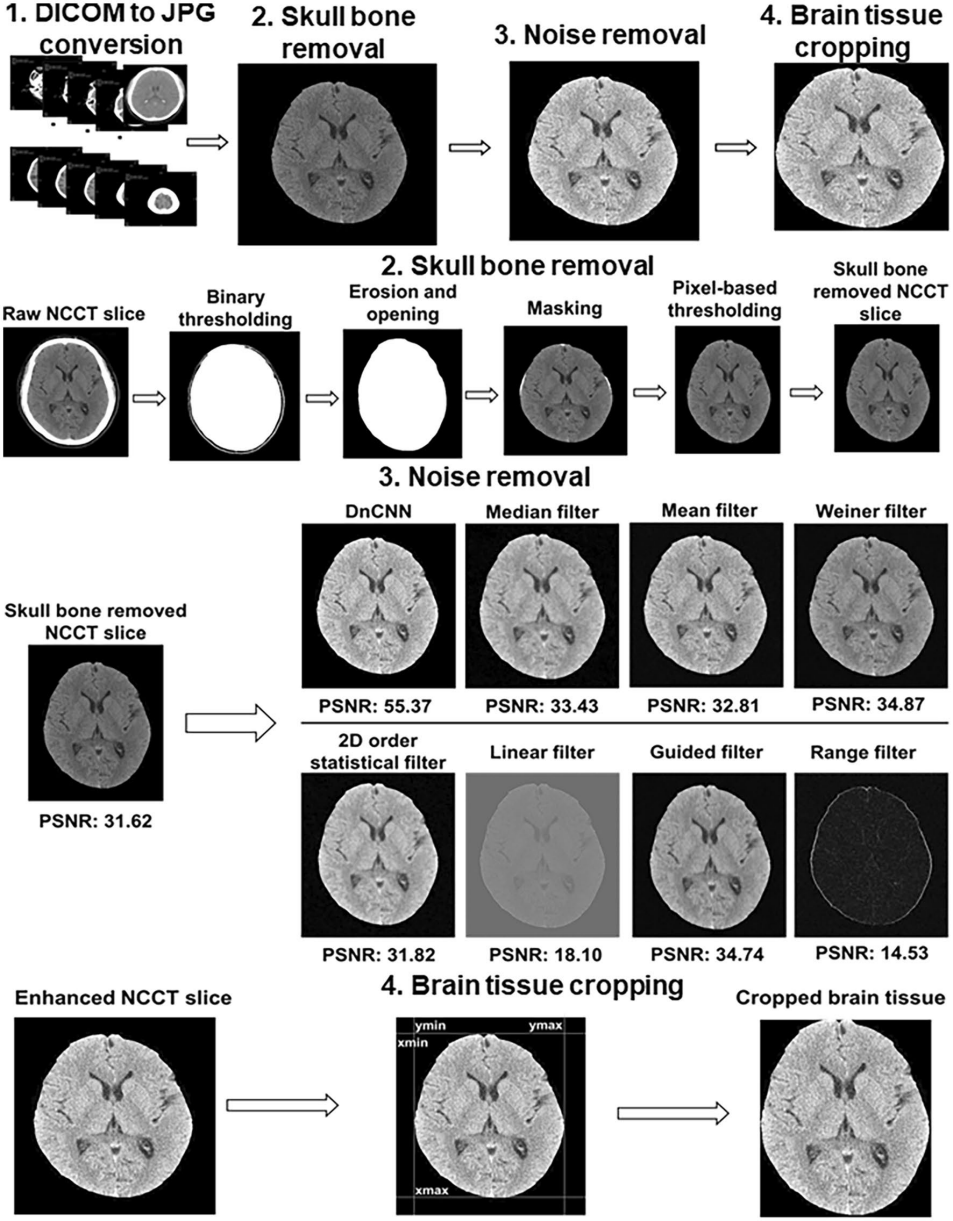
Procedures of NCCT image preprocessing. The upper part shows overall preprocessing steps starting from 2D NCCT slices construction to brain tissue cropping. The next part conveys the intermediate-steps used for skull-bone removal. A comparison of PSNR for different noise removal algorithms are presented in the 3rd part. The final part displays the selection of rectangular non-brain tissue region for cropping of the exact brain tissue part. *NCCT* non-contrast computed tomogram, *2D* two dimensions, *PSNR* peak-to-signal noise ratio.
